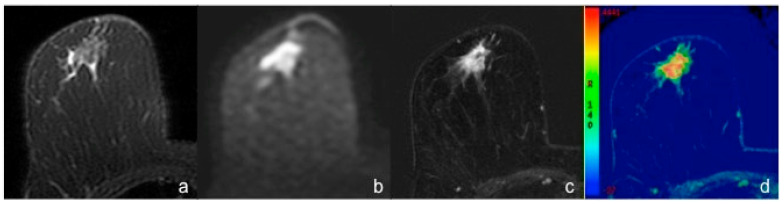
Breast MRI of a 57-year-old woman with IDC of the right breast (Luminal B). (a) Axial fat-suppressed T2-weighted image shows a hypointense irregular mass with spiculated margins and some hyperintense peripheric areas consistent with perilesional edema, in the retroareolar region. (b) Axial DWI image (b value = 1000 s/mm^2^) shows the high signal intensity of the mass, corresponding to a restricted diffusion area. (c) Axial early post-contrast T1-weighted image and the corresponding perfusion color map (d) show a 16 mm irregular mass with spiculated margins and heterogeneous enhancement.
